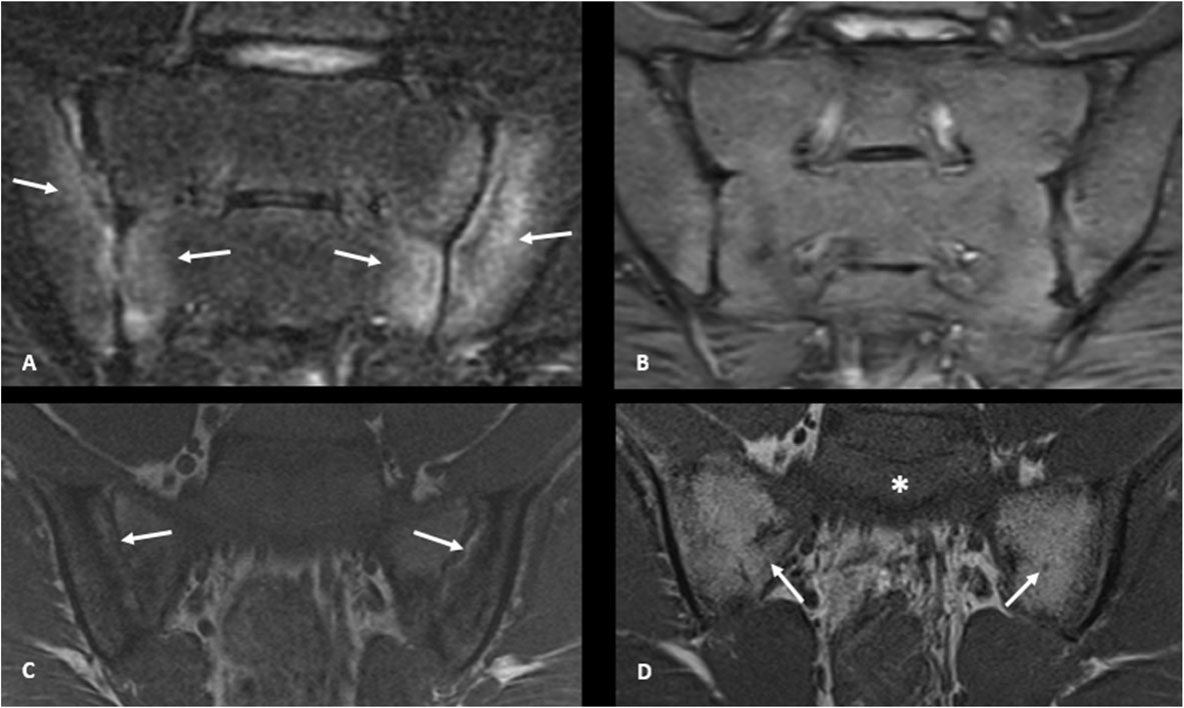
Interval change in SIS and SSS lesions in children. Legend: Coronal oblique STIR images of the sacroiliac joints in a 12-year-old girl (a) and at age 16 years (b). a There is marrow edema along both the iliac and sacral aspects of the sacroiliac joints (arrows), left greater than right. Findings are consistent with active sacroiliitis. On follow-up imaging 4 years later (b), the marrow edema has resolved. Coronal oblique T1-weighted images of the sacroiliac joints in a 15-year-old boy (c) and at age 19 years (d). c There are erosions along both aspects of the iliac bones. In addition, there is high T1-weighted signal within both joints consistent with backfill (arrows). On follow-up imaging 4 years later (d), there is ankylosis of both joints with fat metaplasia, as demonstrated by fatty marrow signal along the fused sacroiliac joints (arrows). Note the normal appearance of the marrow within the upper sacrum demonstrated low signal (*)
